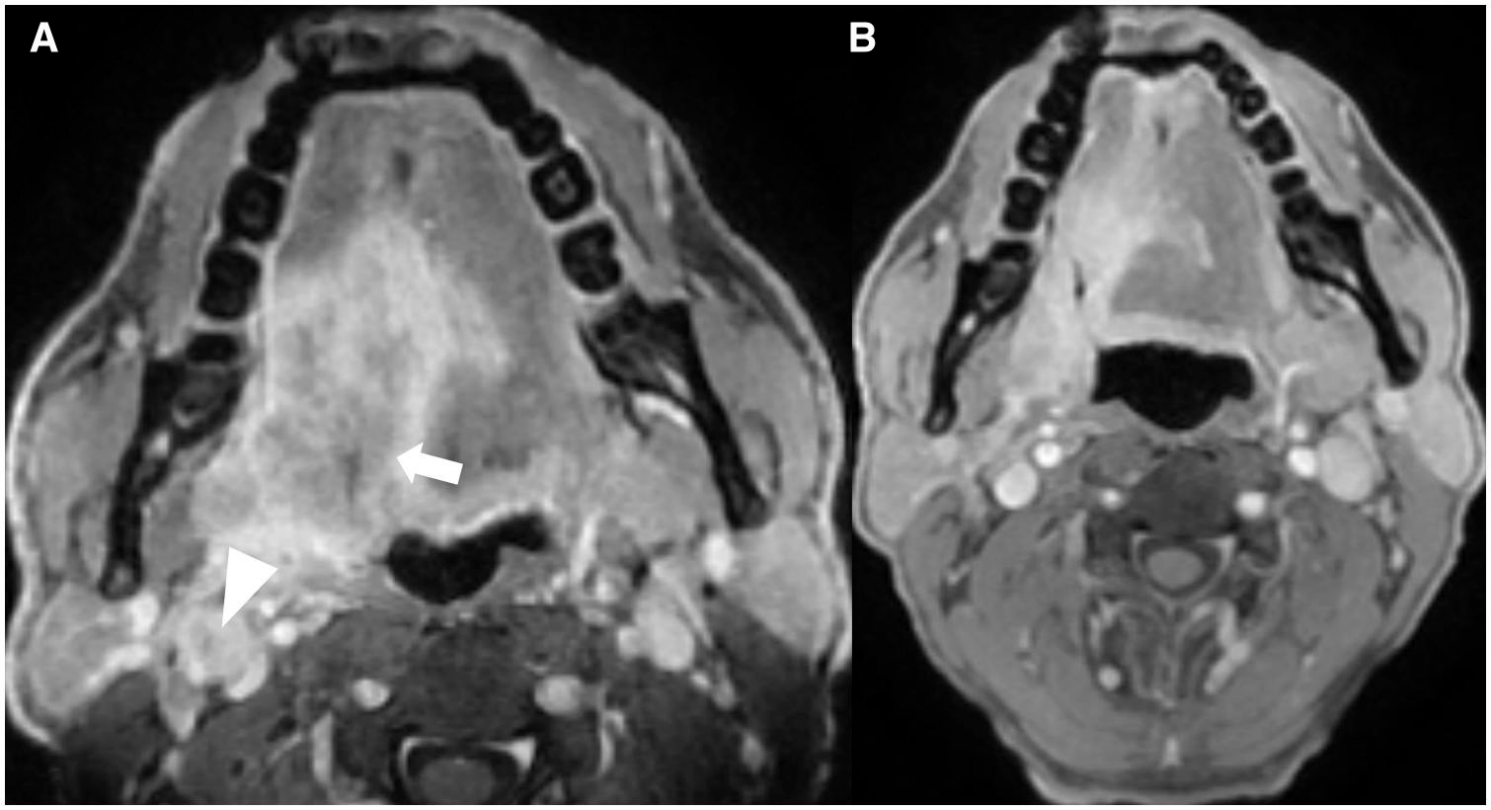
(A) Pre-neoadjuvant chemotherapy (NACT) MRI shows T4bN1 carcinoma right side base of tongue (arrow) with a metastatic right level II node (arrowhead). (B) Post-NACT MRI shows partial response of the primary as well as the node as per RECIST 1.1.
